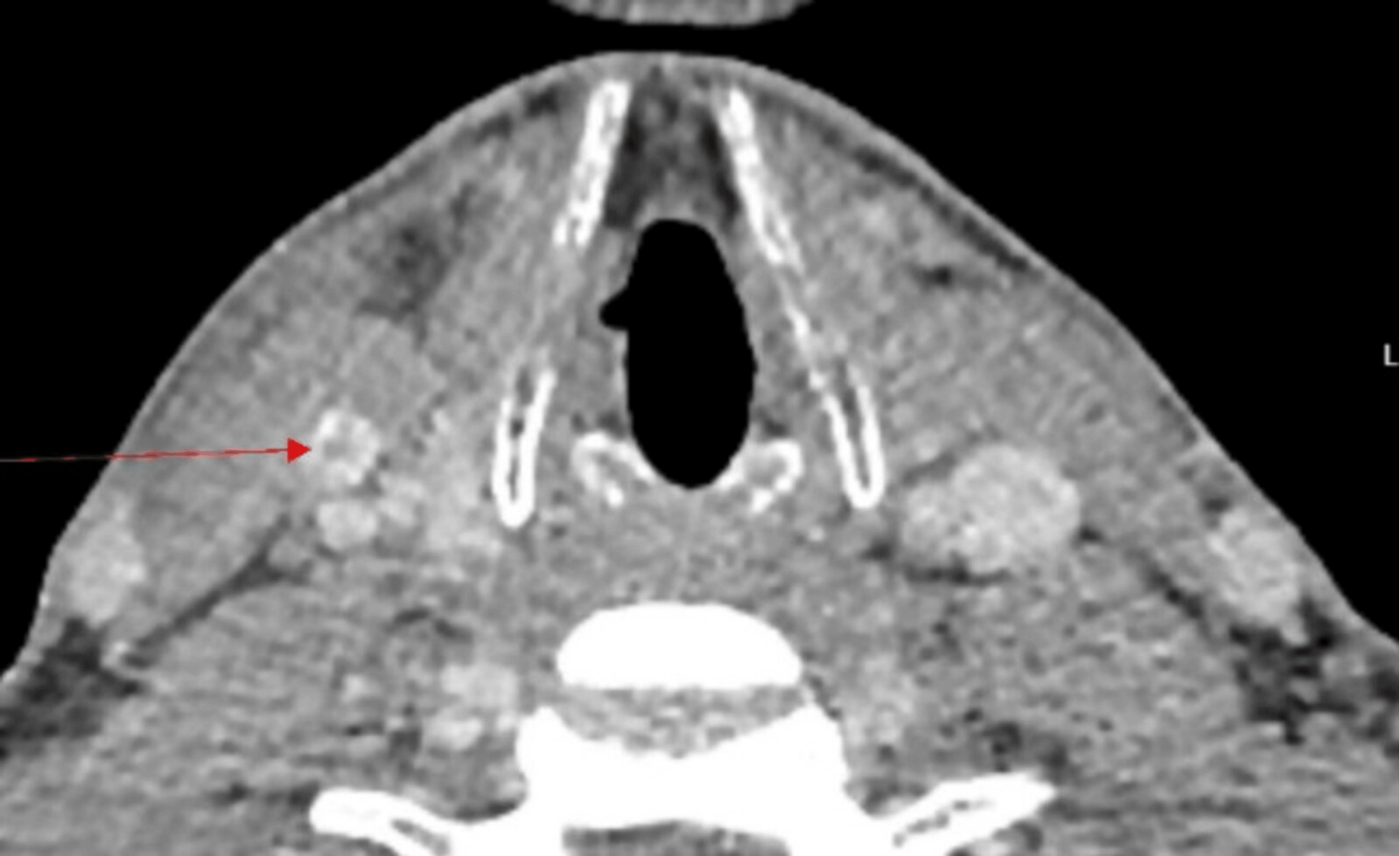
Transverse CT of the neck suggesting right internal jugular vein thrombophlebitis.
